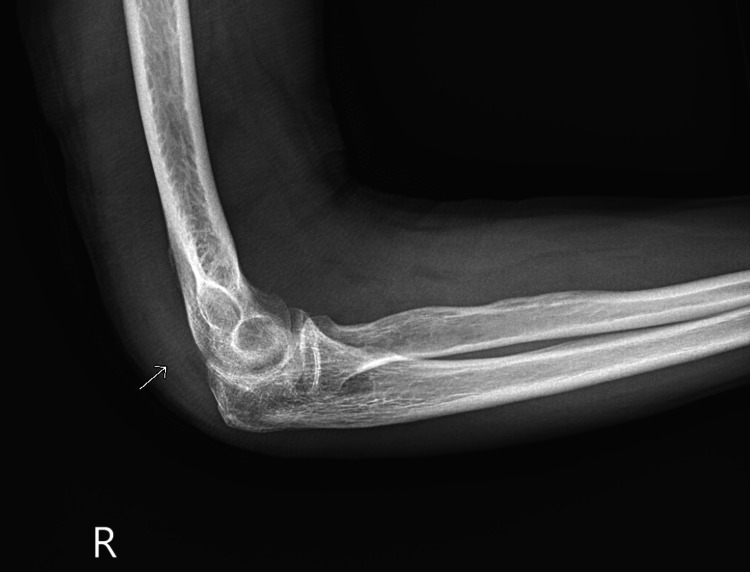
X-ray of the right elbow. Large joint effusion distending the anterior recess. There is a periosteal reaction in the posterior aspect of the humerus and erosions in the coronoid fossa of the distal humerus, creating a scalloped appearance. There is preservation of the joint space and mild periarticular osteopenia.
